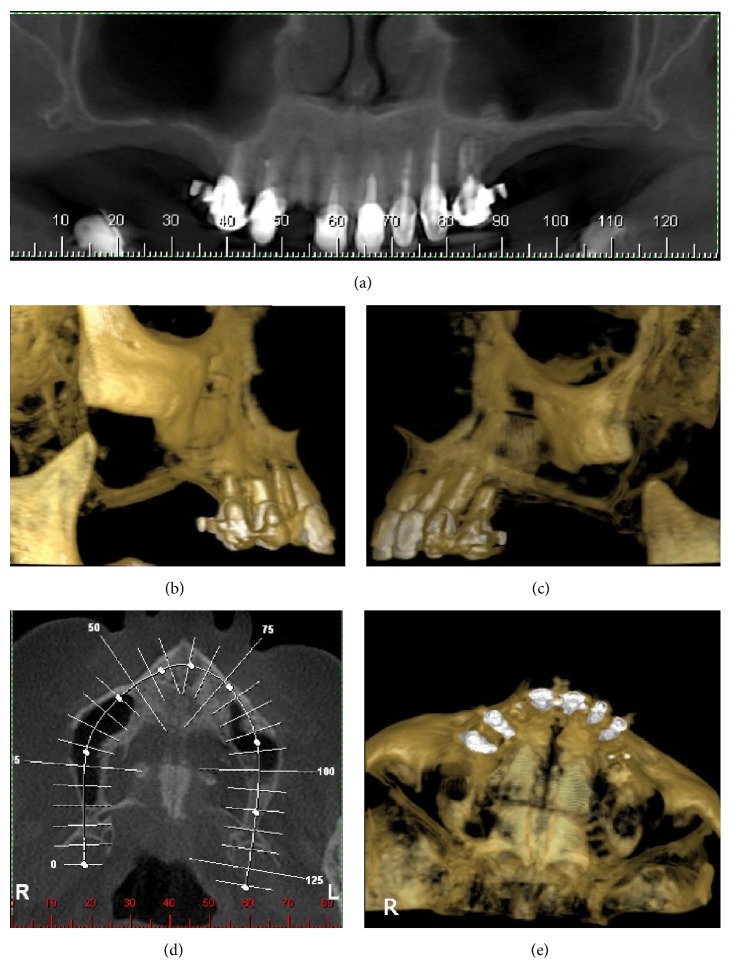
(a) Panoramic view showing a healthy maxillary sinus cavity after 12 months. (b), (c) Tridimensional reconstruction computed tomography scan showing preserved bony structures. (d) and (e) Occlusal slice showing unobstructed maxillary sinus cavities.
